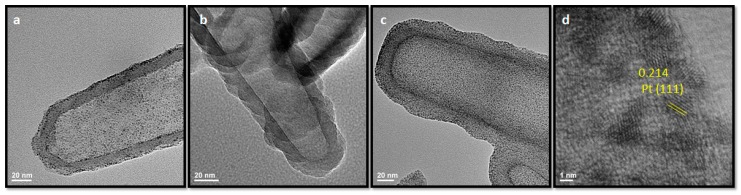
TEM images of SiNTs after incubated in (a) impure cisplatin (~15 wt % platinum (Pt), evaluated by erergy dispersive x-ray analysis (EDX), (b) pure cisplatin (>95%) (~2 wt % Pt, EDX), (c) K_2_PtCl_4_ (50 wt % Pt, EDX)_._ (d) High resolution TEM image showing lattice spacing of Pt species deposited on SiNTs after using K_2_PtCl_4_ as a Pt precursor.
